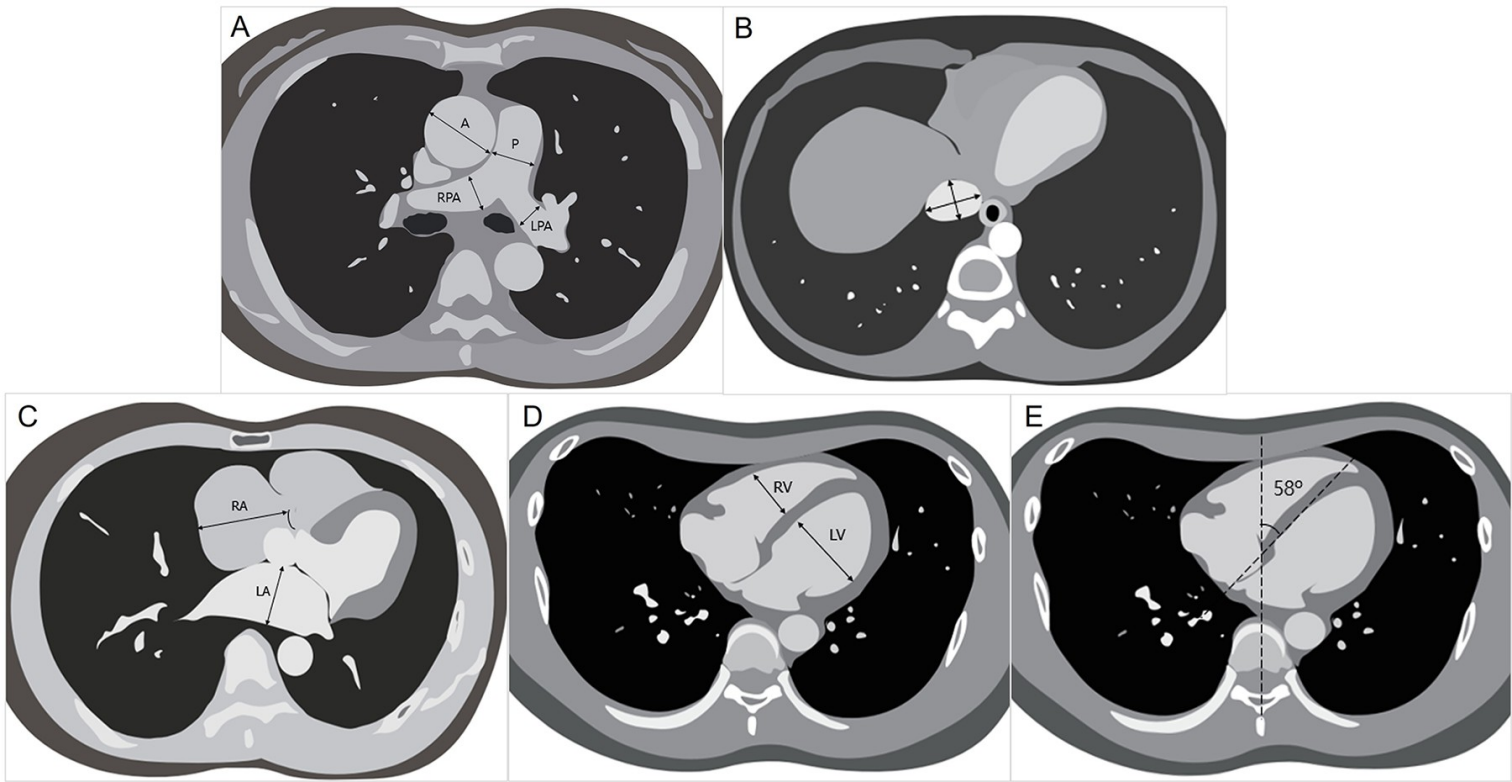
CT imaging parameters. A. The diameter of ascending aorta (A), main pulmonary artery (P), right pulmonary artery (RPA) and left pulmonary artery (LPA). B. Short and long diameter of inferior vena cava. C. Right ventricle (RV) and left ventricle (LV) diameter. D. Right atrium (RA) and left atrium (LA) diameter. E. Septal angle.
